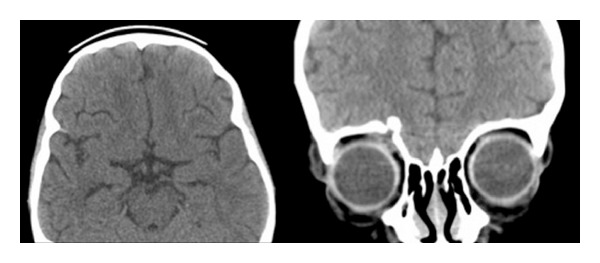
Computed tomography (CT) scans of the cranium demonstrating the resolution of frontal edema and abscess 8 weeks after treatment.
